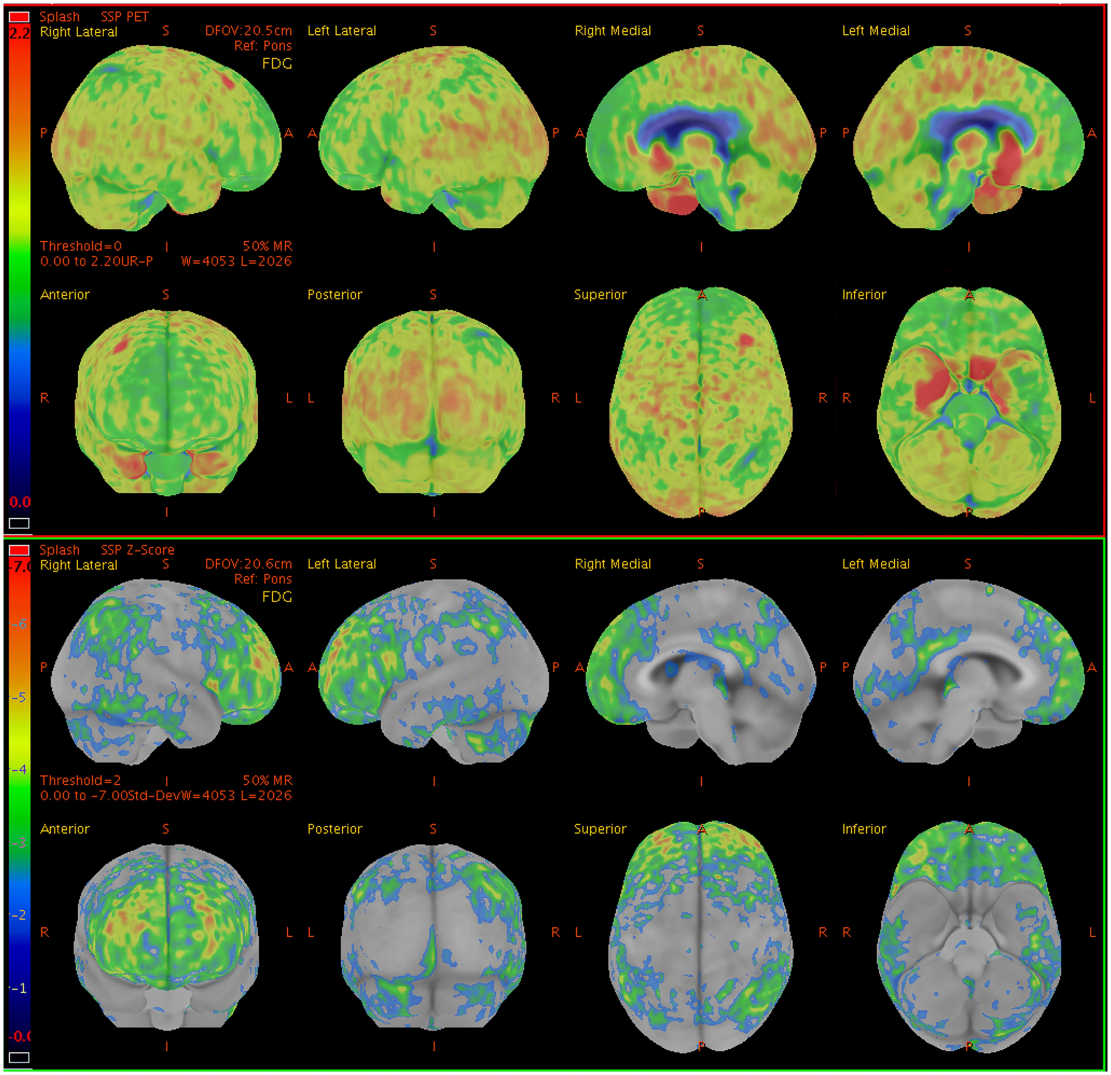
Sample brain ^18^F-FDG PET images from a 60-y-old man presenting with seizures, and memory impairment. The brain ^18^F-FDG PET *z*-score maps demonstrate significant hypometabolism in the lateral/medial prefrontal lobe (R, L), posterior cingulate gyrus (R, L), precuneus (R, L), superior parietal lobe (R), inferior parietal lobe (R, L), lateral occipital lobe (R, L), primary visual cortex (R, L), and lateral temporal lobe (R) relative to metabolic activity in the pons. In contrast, significant hypermetabolism is visible in the medial temporal lobe (R, L). Color bar represents the scale of the *z*-scores. ^18^F-FDG PET, ^18^F-fluorodeoxyglucose positron emission tomography; R, right side; L, left side.
